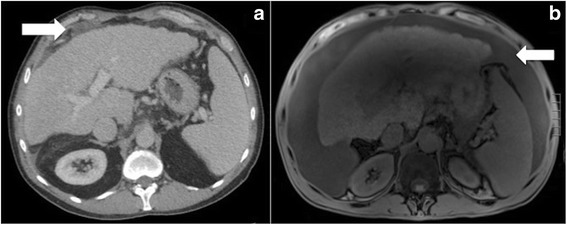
a Preoperative computed tomography scan of a patient with pseudomyxoma peritonei from low-grade appendiceal mucinous neoplasm with cirrhosis. Arrow shows mucinous implants on the peritoneal surface of the right hemi-diaphragm. b Magnetic resonance imaging scan at 6 months postoperatively showing diffuse ascites. This fluid was removed by paracentesis and was serous. Cytology was negative for mucin or malignant cells, and the ascites was resolved with sodium restriction and diuresis
